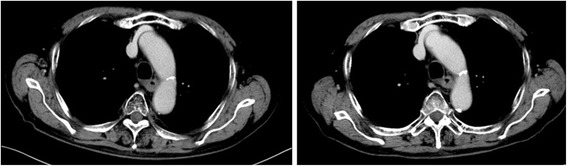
Computed tomographic images obtained 16 (right figure) and 25 months (left figure) after completion of radiotherapy for esophageal basaloid squamous cell. There has been no evidence of recurrence or metastasis since the initiation of this treatment
